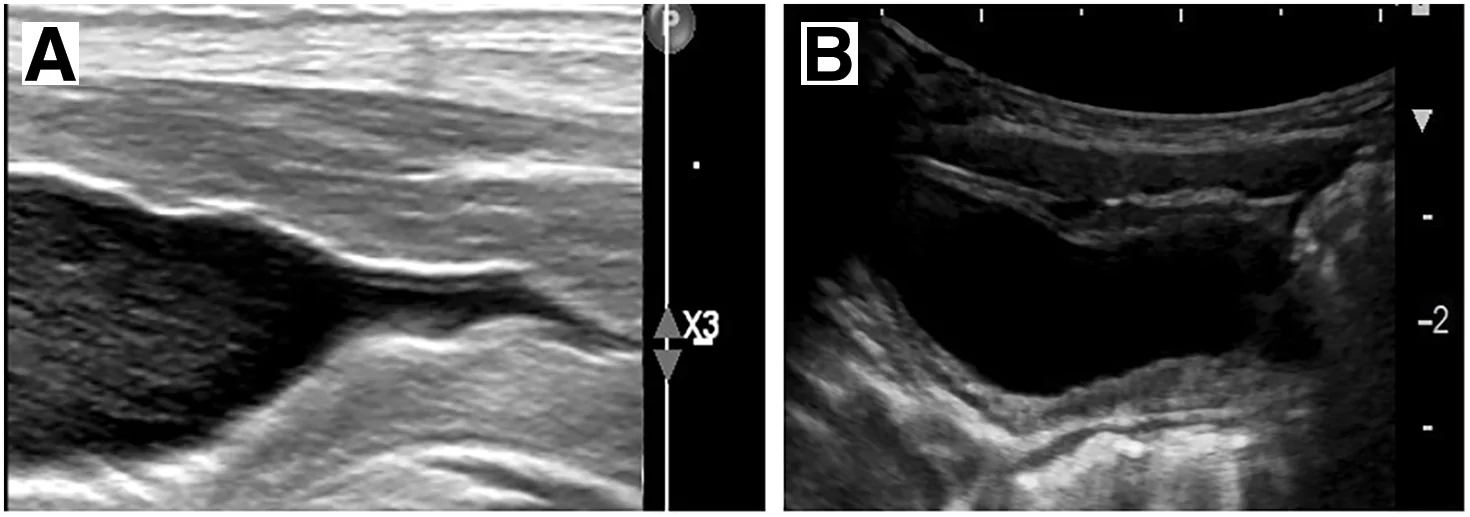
Bladder ultrasonography imaging. (A) Postnatal day 1: no bladder-wall thickening. (B) Most recent evaluation (28 months of age): bladder-wall thickness approximately 6 mm without trabeculation.
